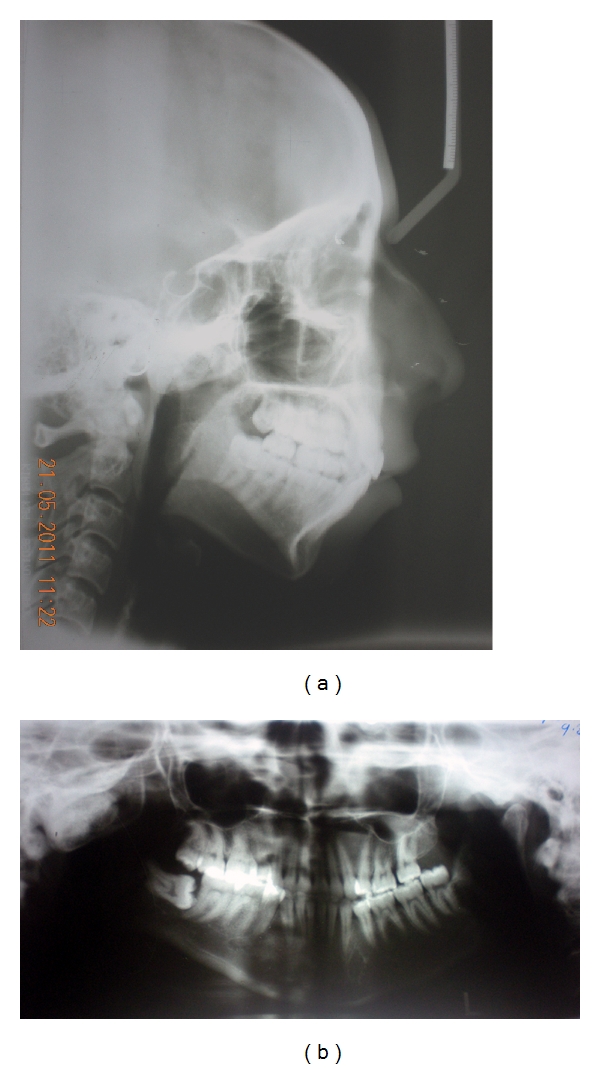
(a)-(b) radiographs after completion of orthodontic treatment and before genioplasty.
